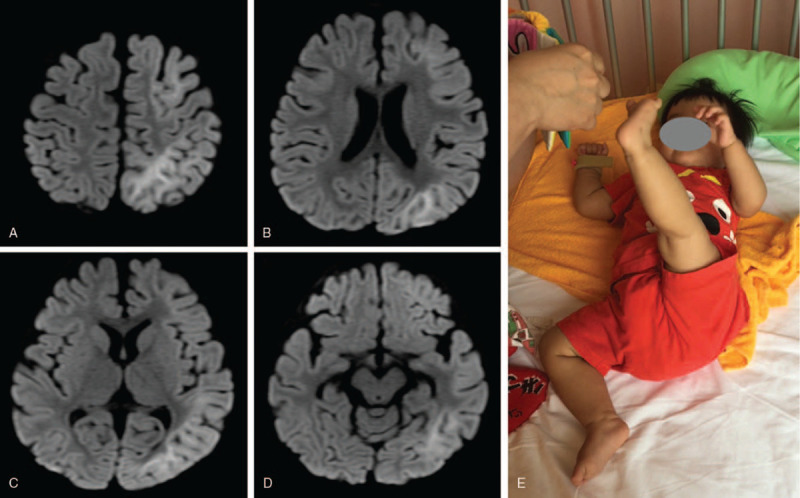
MRI and physical findings of the patient. A-D: Brain MRI on day 4. BTA pattern was seen in the left cerebral hemisphere on DWI. E: Photograph at the start of rehabilitation. The patient presented severe right hemiplegia. The response to the toy was good. BTA = bright tree appearance, DWI = diffusion-weighted image, MRI = magnetic resonance imaging.
